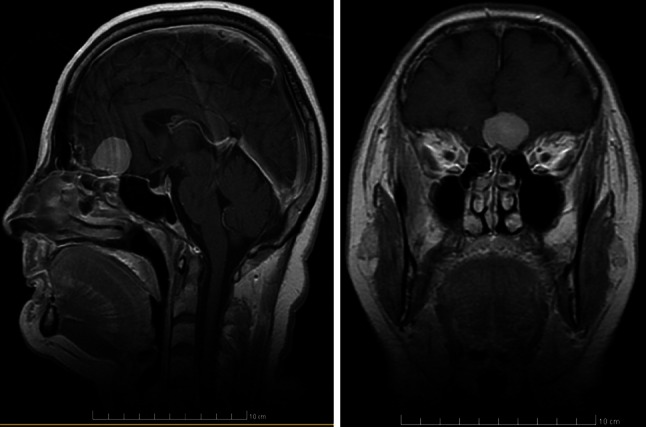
MRI (T2). Female patient aged 52—case number 1, history of anosmia for 1 year, seizures for 2 years
